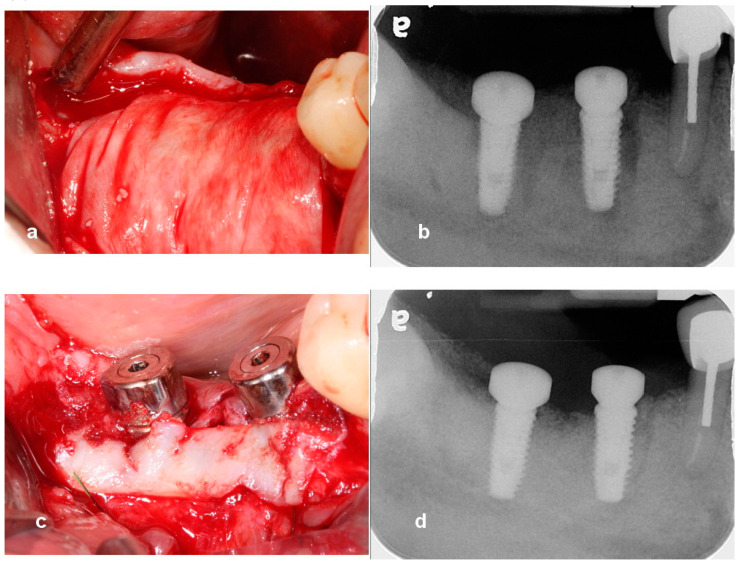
(a) A collagen barrier was used to cover the grafted site. Bone tacks were not used to stabilize the membrane. (b) A radiograph taken immediately after surgery shows bone graft around healing abutments and implants. (c) The uncovering was carried out after 5 months of healing. Suture-less free gingival graft was performed to attach gingiva around implant-supported restoration. (d) A periapical radiograph reveals successful ridge augmentation after 5 months of healing.
